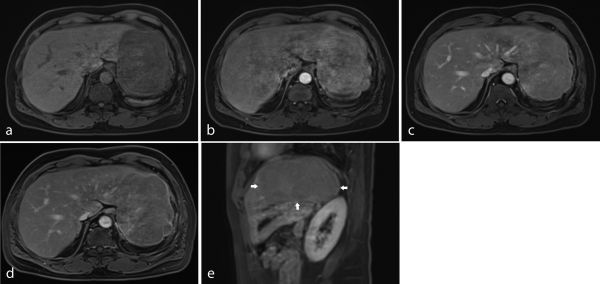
Pre-contrast *T*_1_ weighted fat-suppression image shows a hypointense mass at the left hepatic lobe (a). Dynamic contrast-enhanced MRI shows heterogeneous enhancement of the mass at the left hepatic lobe in the arterial phase (b). The mass becomes more homogeneous on portal venous and delayed images (c, d). Sagittal image acquired during the delayed phase shows a homogeneous mass (outlined by arrows), which appears relatively hypointense to the liver parenchyma (e).
